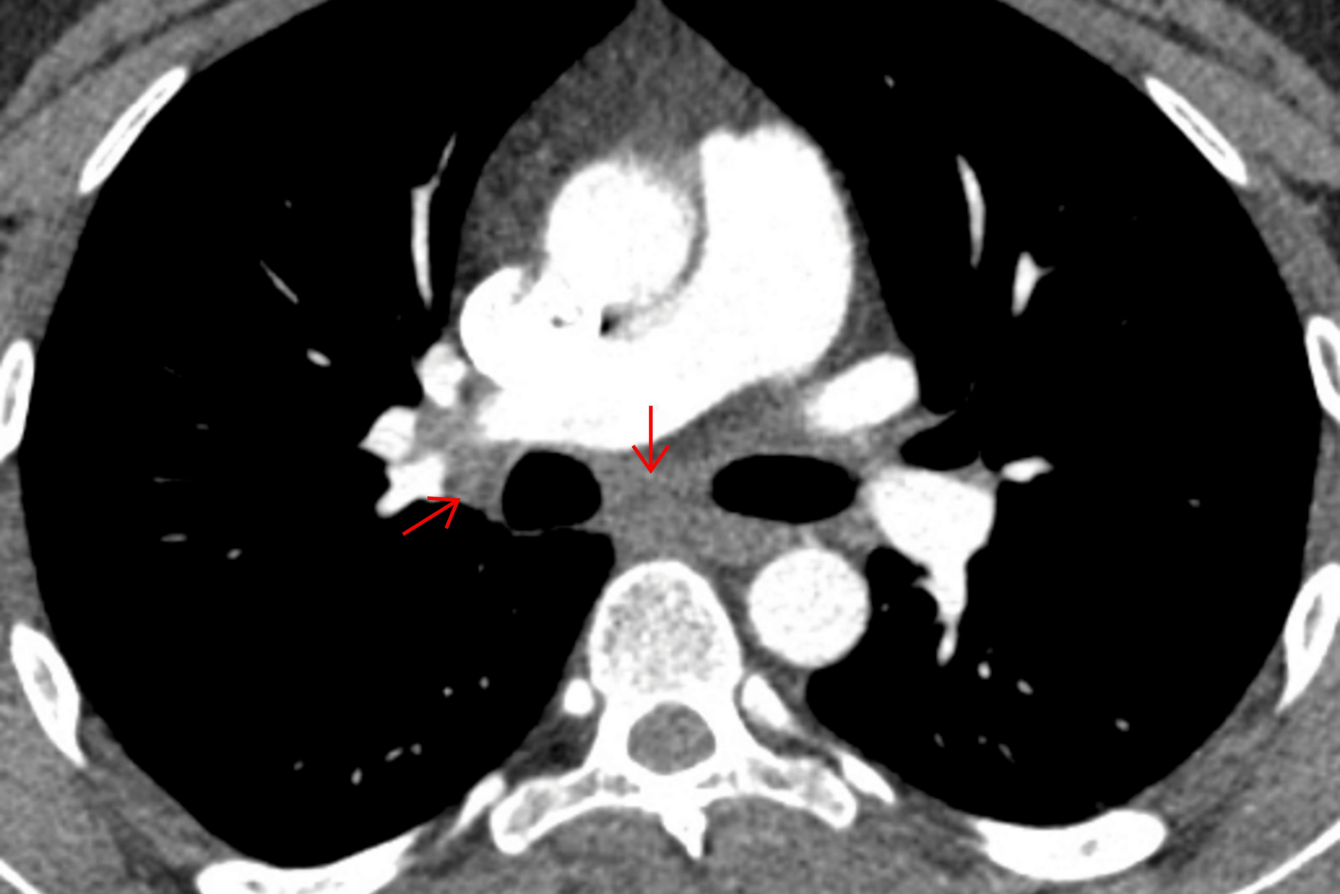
CT thorax showing complete resolution of right hilar and subcarinal lymph node CT: computed tomography
